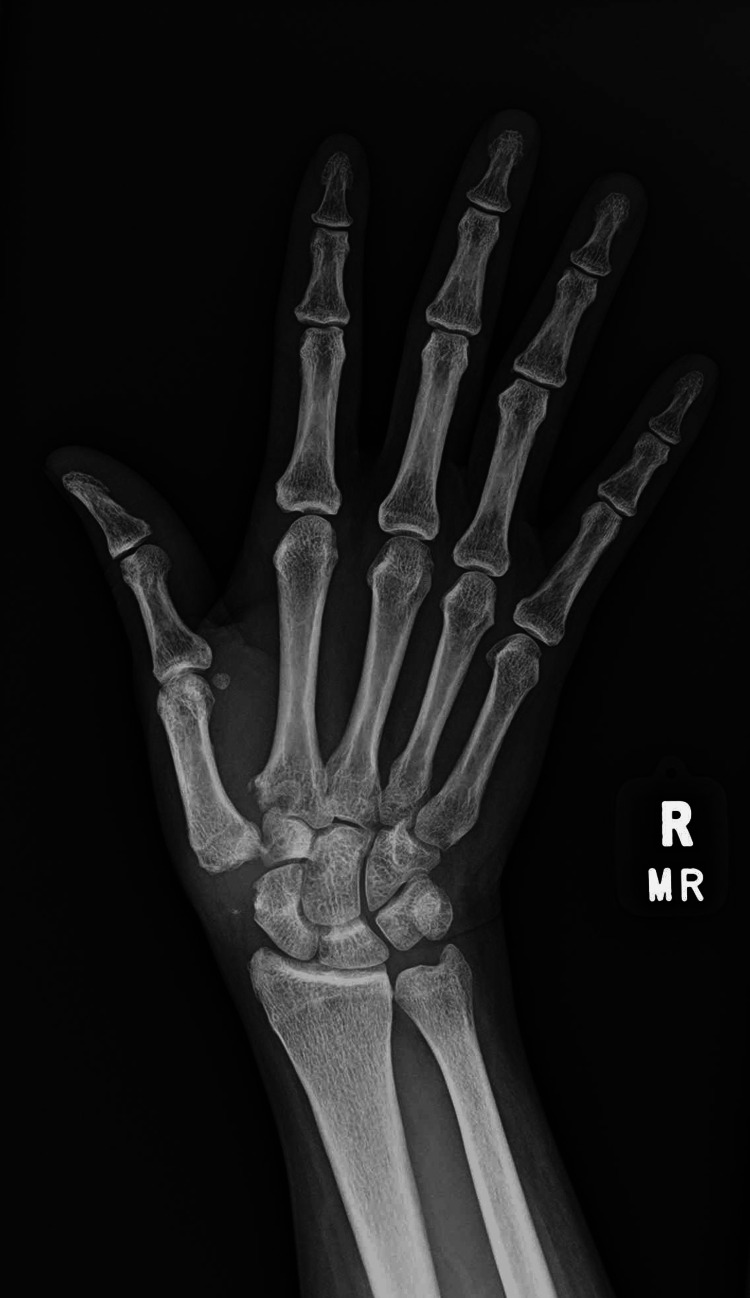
Posteroanterior radiograph of the right hand demonstrating a complete collapse of the first carpometacarpal joint. There is marked subsidence of the first metacarpal.
